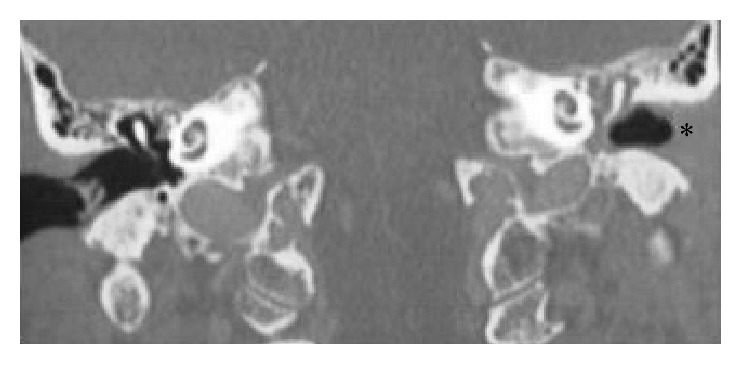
CT imaging depicting otitis media of the left ear with external ear canal obstruction (*∗*) due to the cystic lesion.
